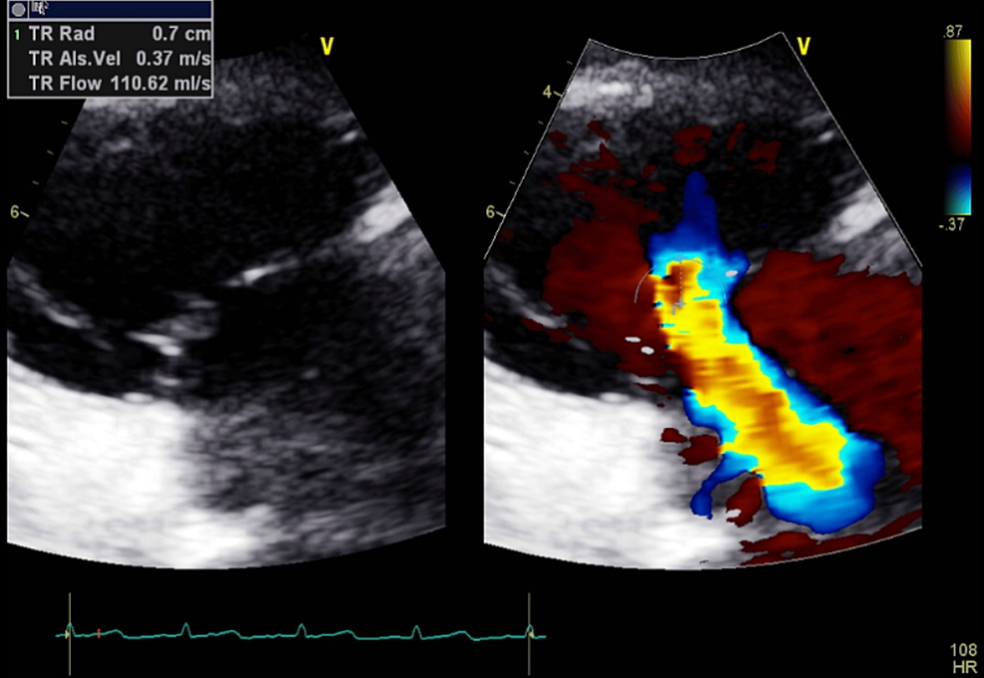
Transthoracic echocardiography post-pericardiocentesis - image 6 Transthoracic echocardiography post-pericardiocentesis; apical 4-chamber view measuring TR radius at 0.7 cm and TR flow at 119.62 ml/s, indicative of severe tricuspid regurgitation
